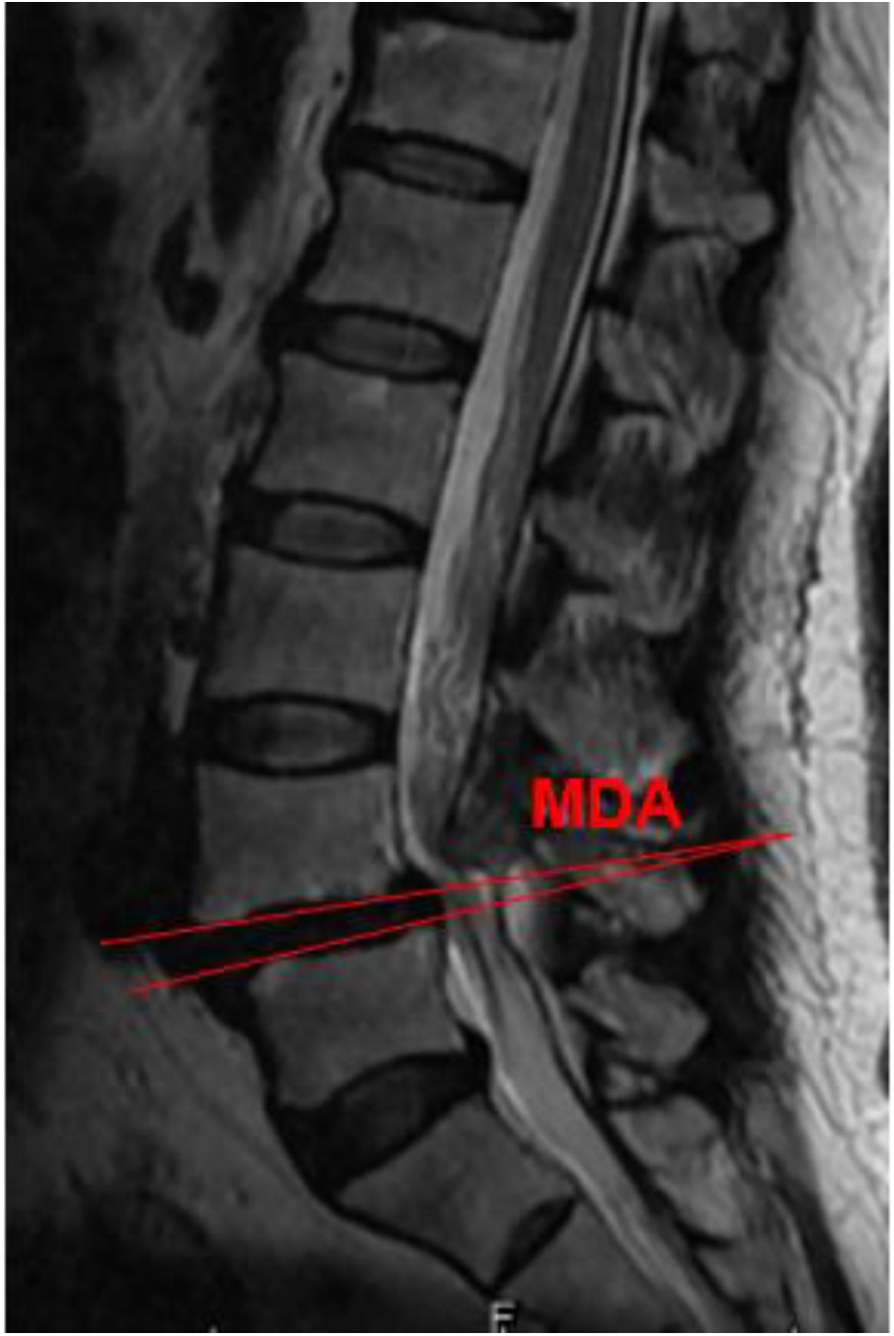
L4 was grade I spondylolisthesis, and MDA was the angle between L4 and L5 on MRI.
